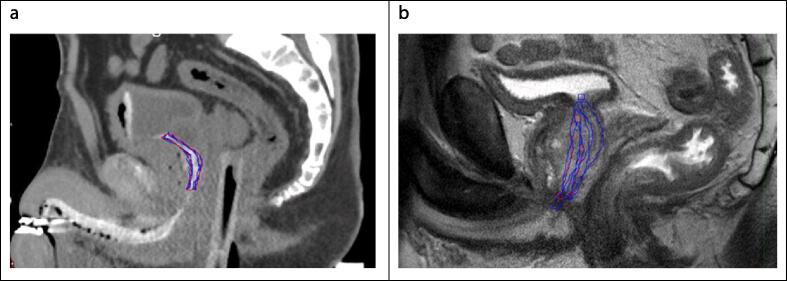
Sagittal example dataset with observer urethra contours (blue line) and reference contour generated based on STAPLE (red line) on CT urethrogram (a), and mpMRI (b). (For interpretation of the references to colour in this figure legend, the reader is referred to the web version of this article.)
